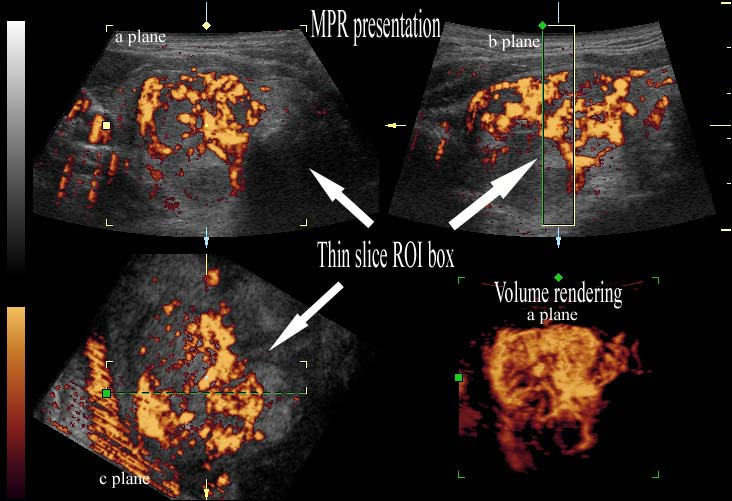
Three-dimensional power Doppler ultrasound of papillary cancer with high density of vessels. Multiplanar reformation (MPR) mode presentation and thin-slice volume rendering (lower right corner). The density of the vessels is in the highest range: 4 = (76-100%) of area of the nodule; ROI - region of interest.
